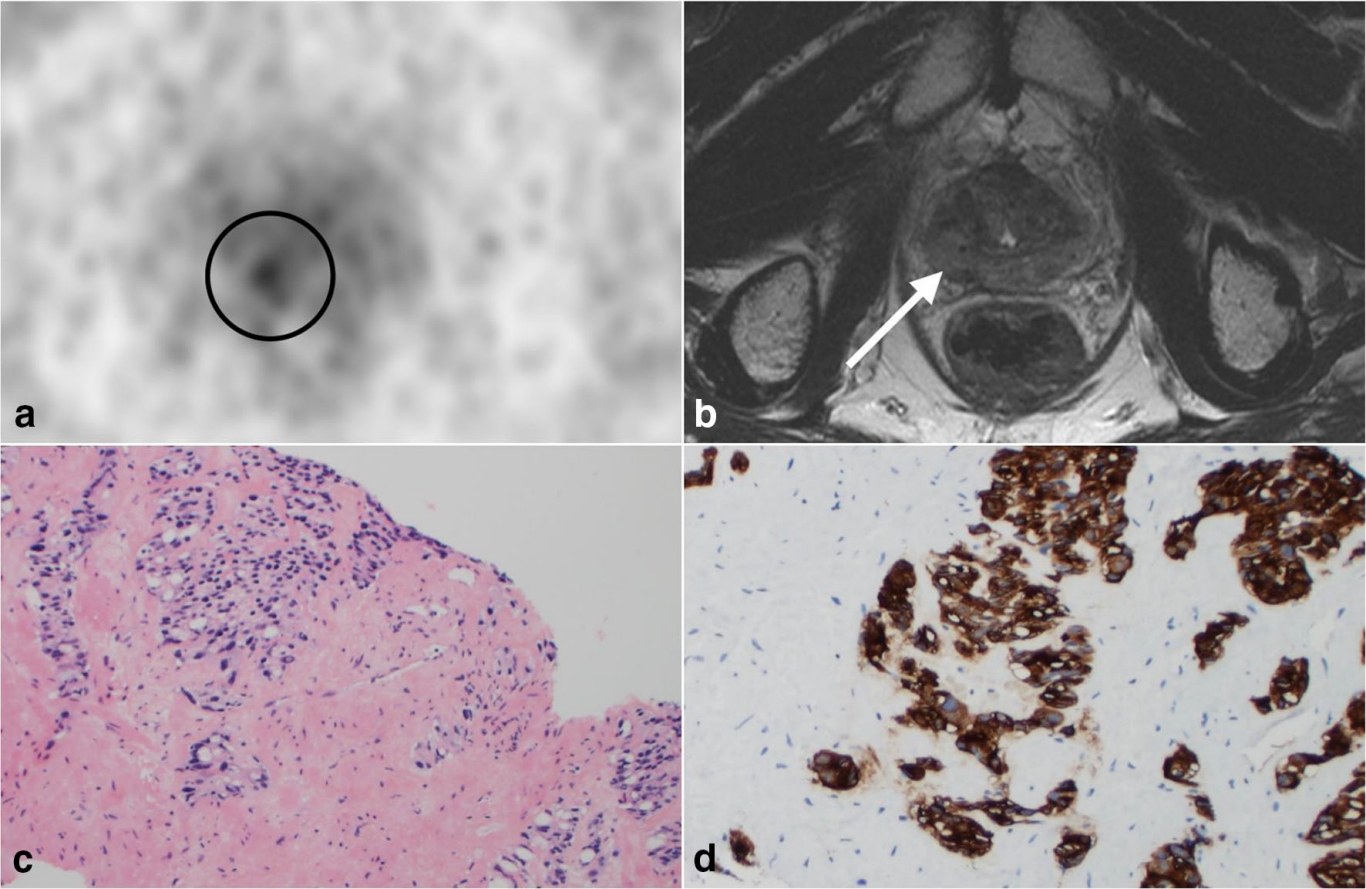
74-year-old man post-radiotherapy performed 10 months prior with biochemical recurrence who had focal uptake on the right peripheral zone of the prostate (a, circle) (PSA 7.2 ng/mL, MRI FP 7). Neither T2 weighted imaging (b, arrow), dynamic contrast enhanced (DCE) imaging or diffusion weighted imaging demonstrated a focal lesion to correlate with the focal uptake (SUV_max_ 12.5) seen on [^68^Ga]Ga-PSMA-11. Trans-anal ultrasound guided core needle biopsy was obtained from the region of uptake. Pathology demonstrates tumor cells with significant treatment effect (c) and marked PSMA expression (d). There was no evidence of PSMA expression in vasculature
